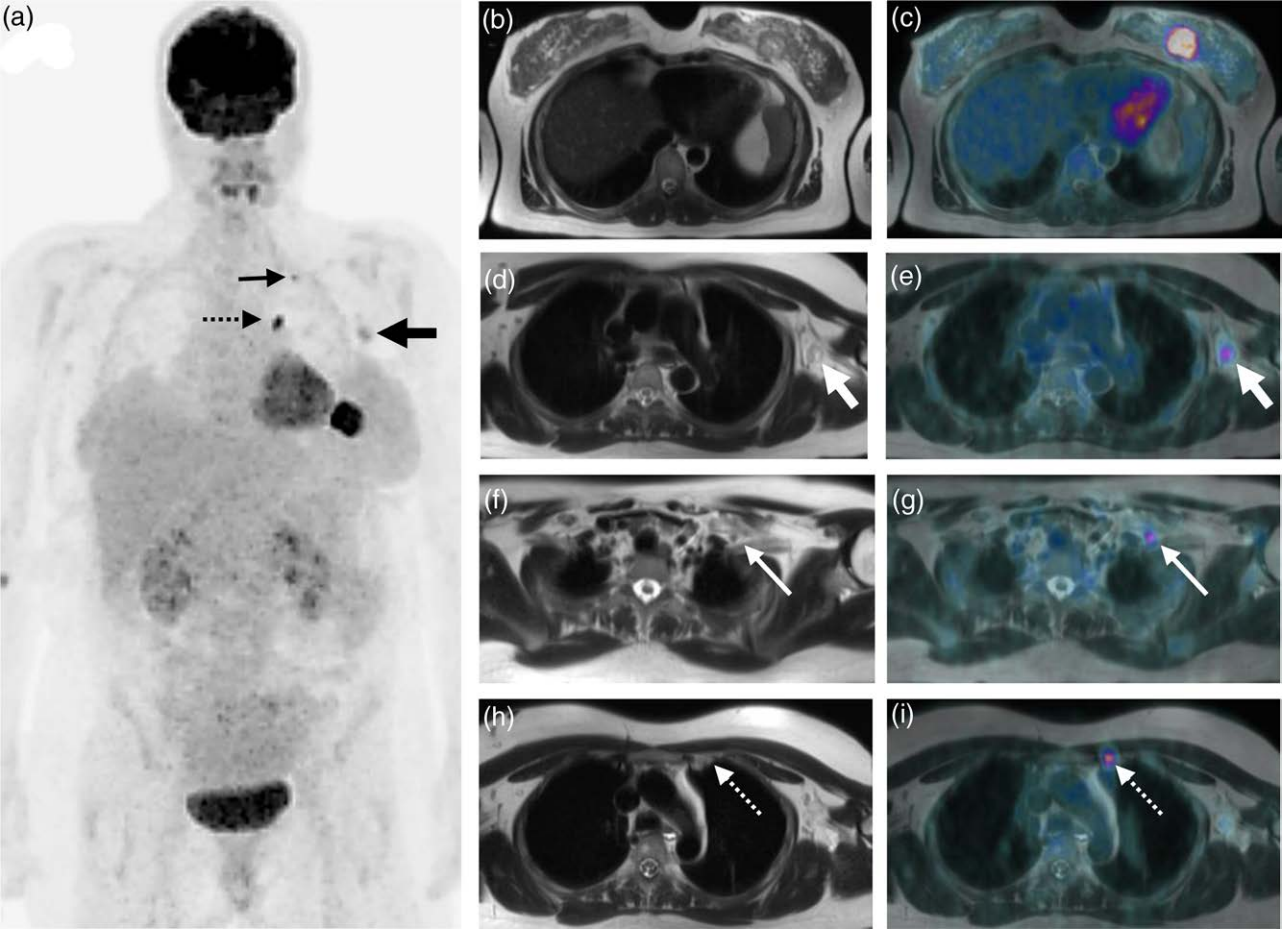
Patient #3. (a) Maximum intensity projection FDG-PET image depicting a left breast malignancy associated with an extensive locoregional nodal disease (arrows). T2-weighted MR images (b,d,f,h) and fused FDG-PET(-MR) images (c,e,g,i) show a large FDG-avid malignant process in the upper inner quadrant of the left breast (b,c). There are FDG-avid subcentimetre left axillary (thick arrow) (d,e), left supraclavicular fossa (thin arrow) (f,g), and left internal mammary chain (dotted arrow) (h,i) lymph node metastases, respectively.
